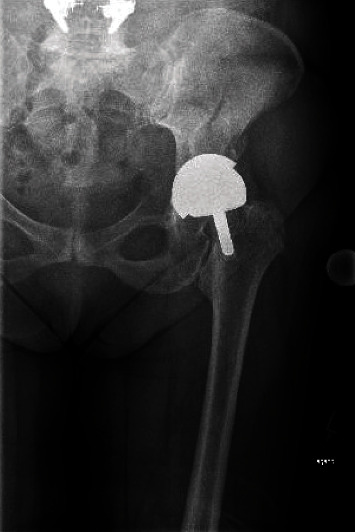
Initial X-ray of the fracture. After a stumble fall, the patient presented herself to an orthopedist. An anteroposterior X-ray of the pelvis was conducted and showed a fracture of the pelvis with a dislocation of the prosthesis.
